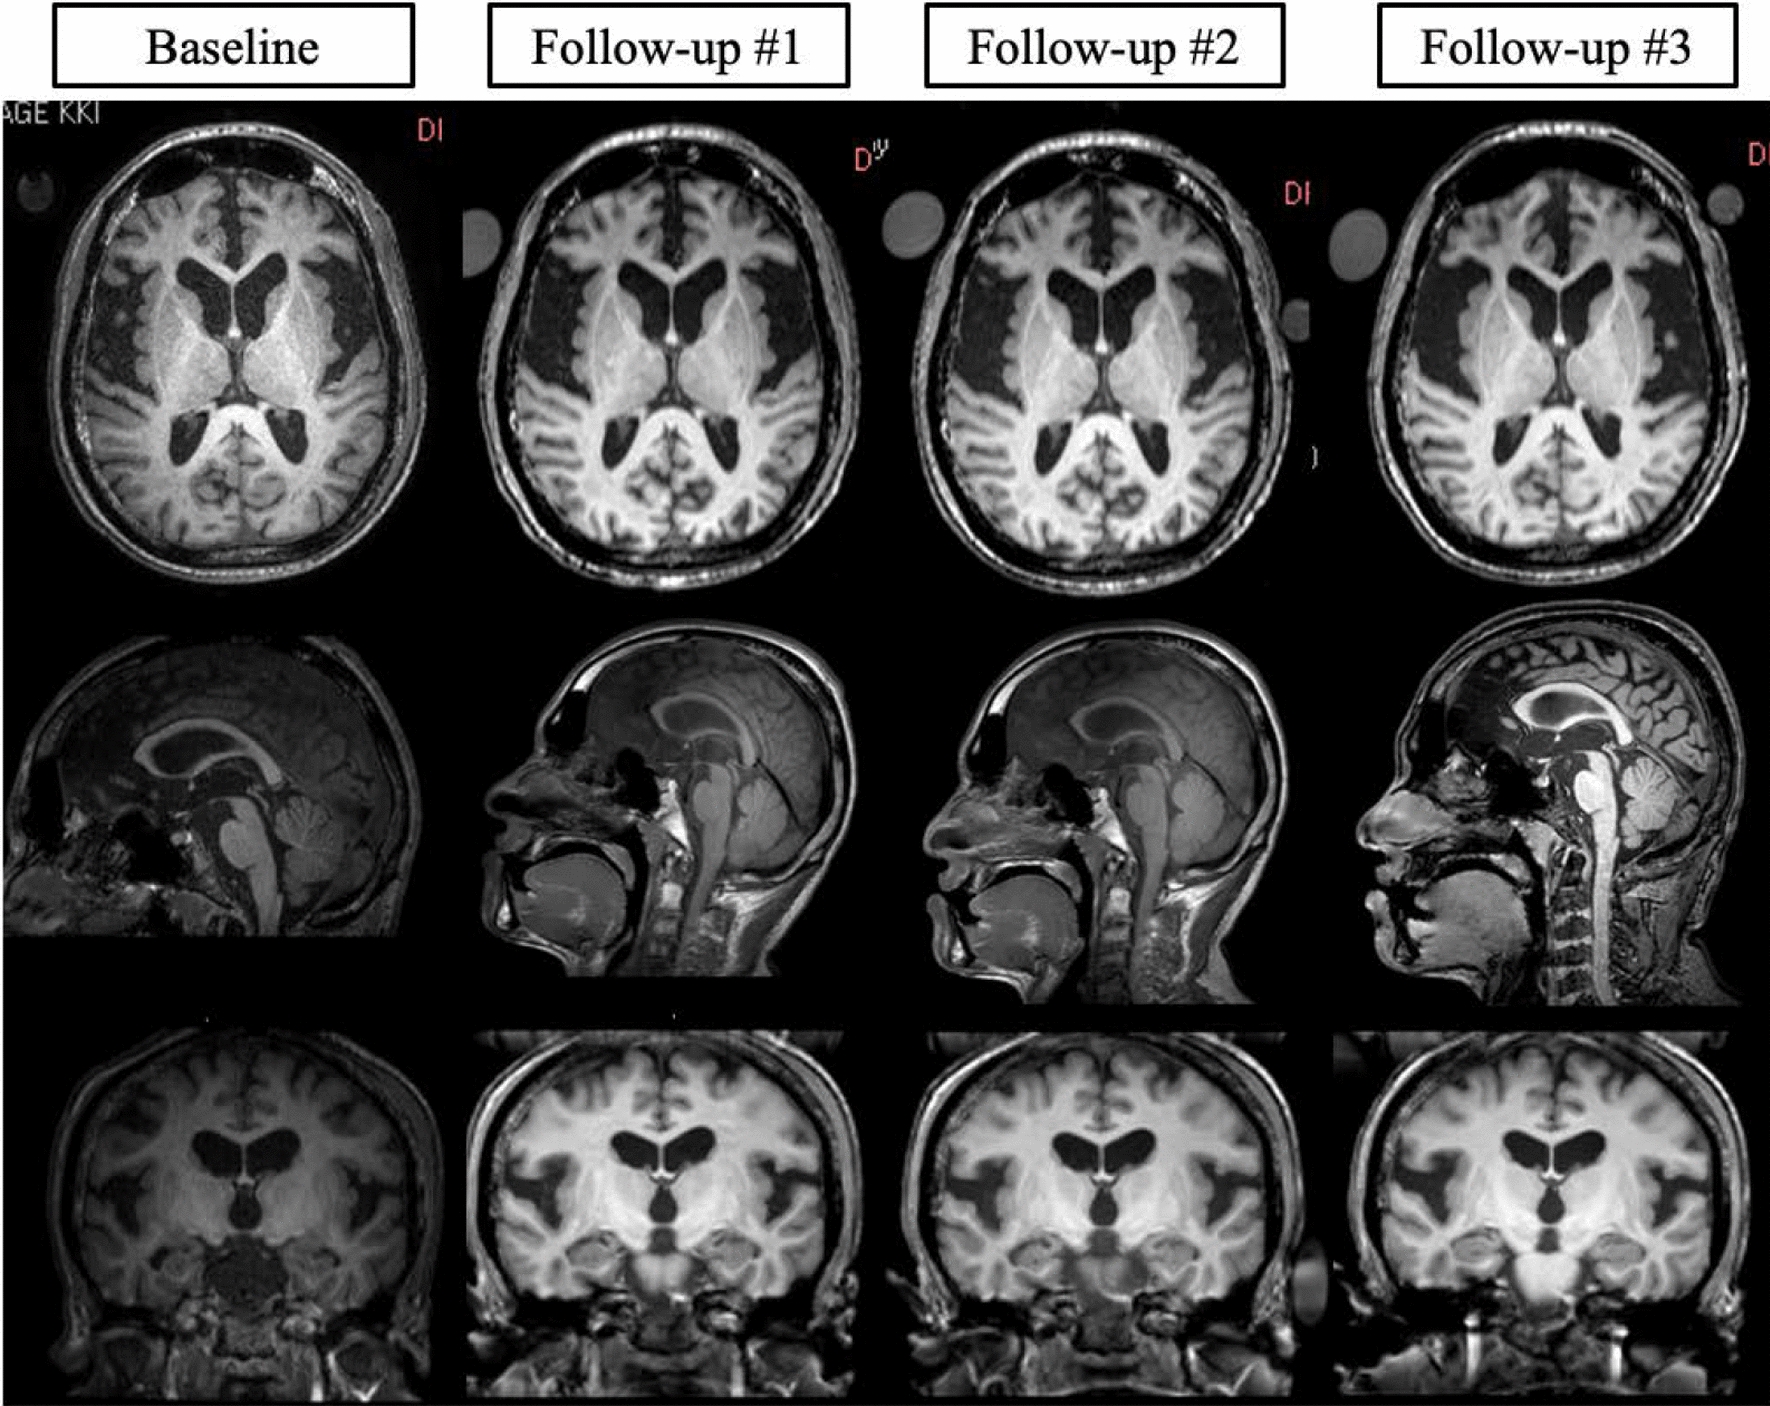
Longitudinal magnetic resonance imaging of patient H. Highlighting baseline cerebrum atrophy and longitudinal stability. Patient H was rated a CGI-C = 4 (No Change) at all three follow-up visits when compared to baseline
